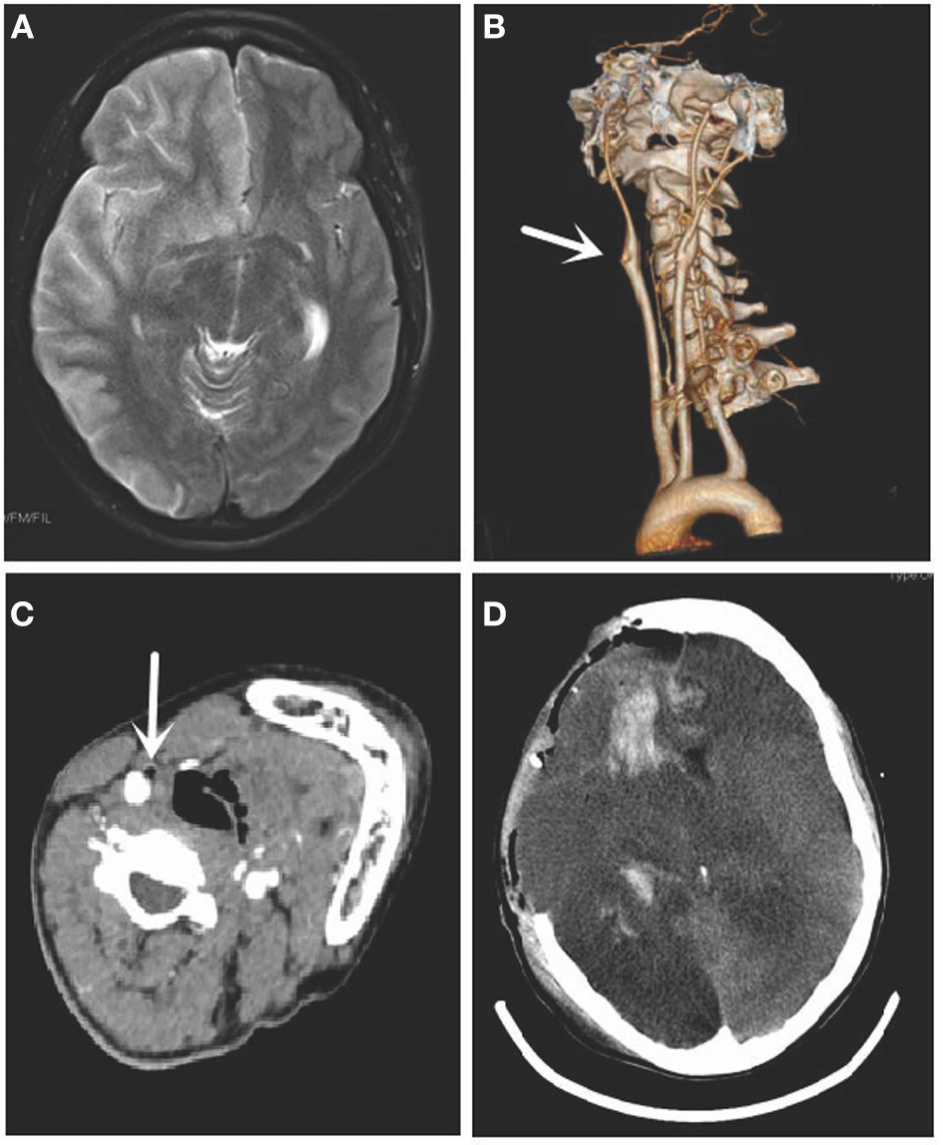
Magnetic resonance imaging (MRI), cervical computed tomography angiography (CTA), and computed tomography (CT) of case 1. (A) The cranial MRI showed acute infarction in the territory of the right internal carotid artery. (B) CTA showed occlusion of the right external carotid artery (arrow). (C) Cross-section indicated fat emboli within the lumen of the right external carotid artery (arrow). (D) Cranial CT showed the right swelling hemisphere and incomplete skull after decompressive craniectomy.
